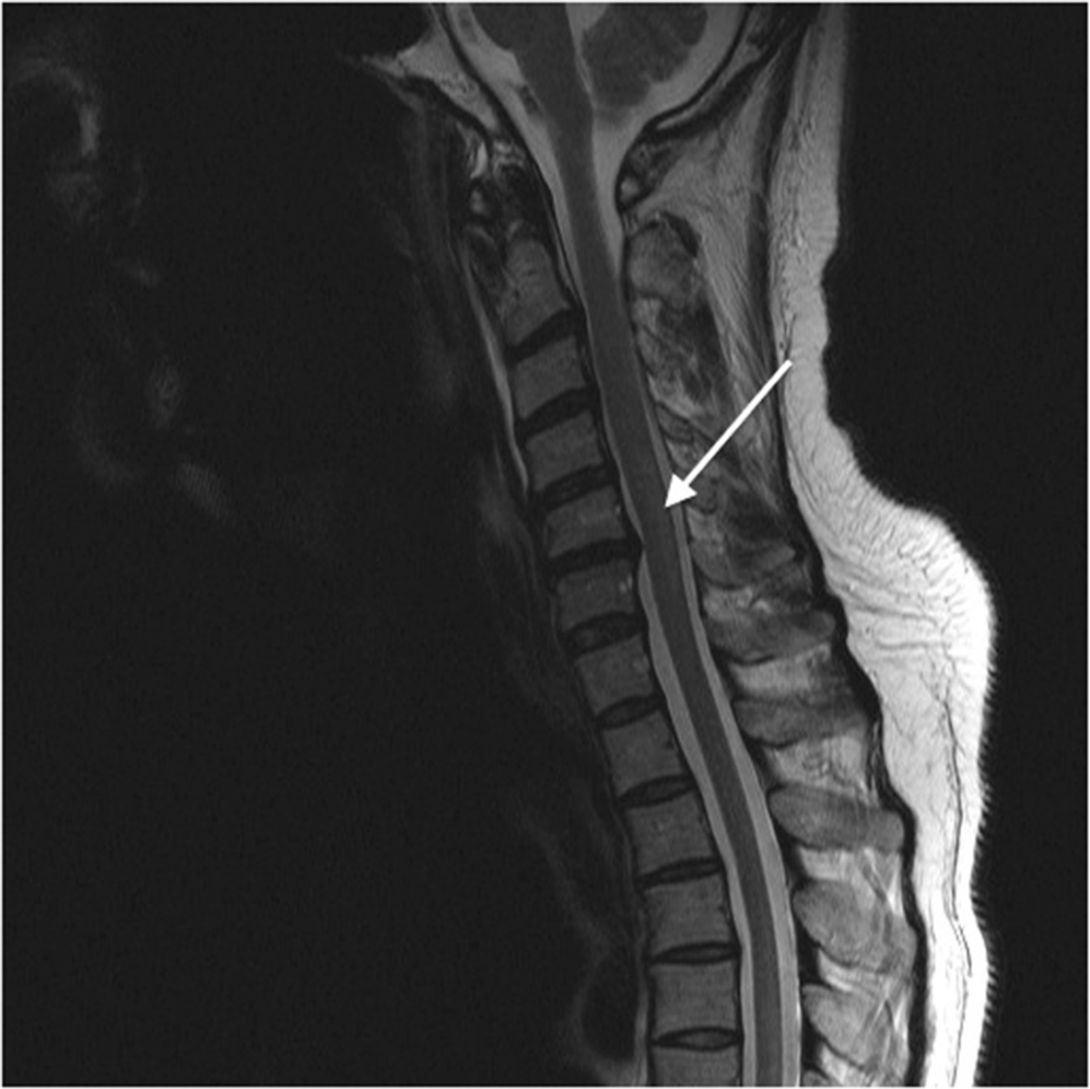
Magnetic resonance imaging scan of the patient’s spine. This sagittal view of the patient’s cervical and thoracic spine shows an area of hyperintensity (indicated by the arrow) along the dorsal column, consistent with demyelination due to vitamin B_12_ deficiency.
